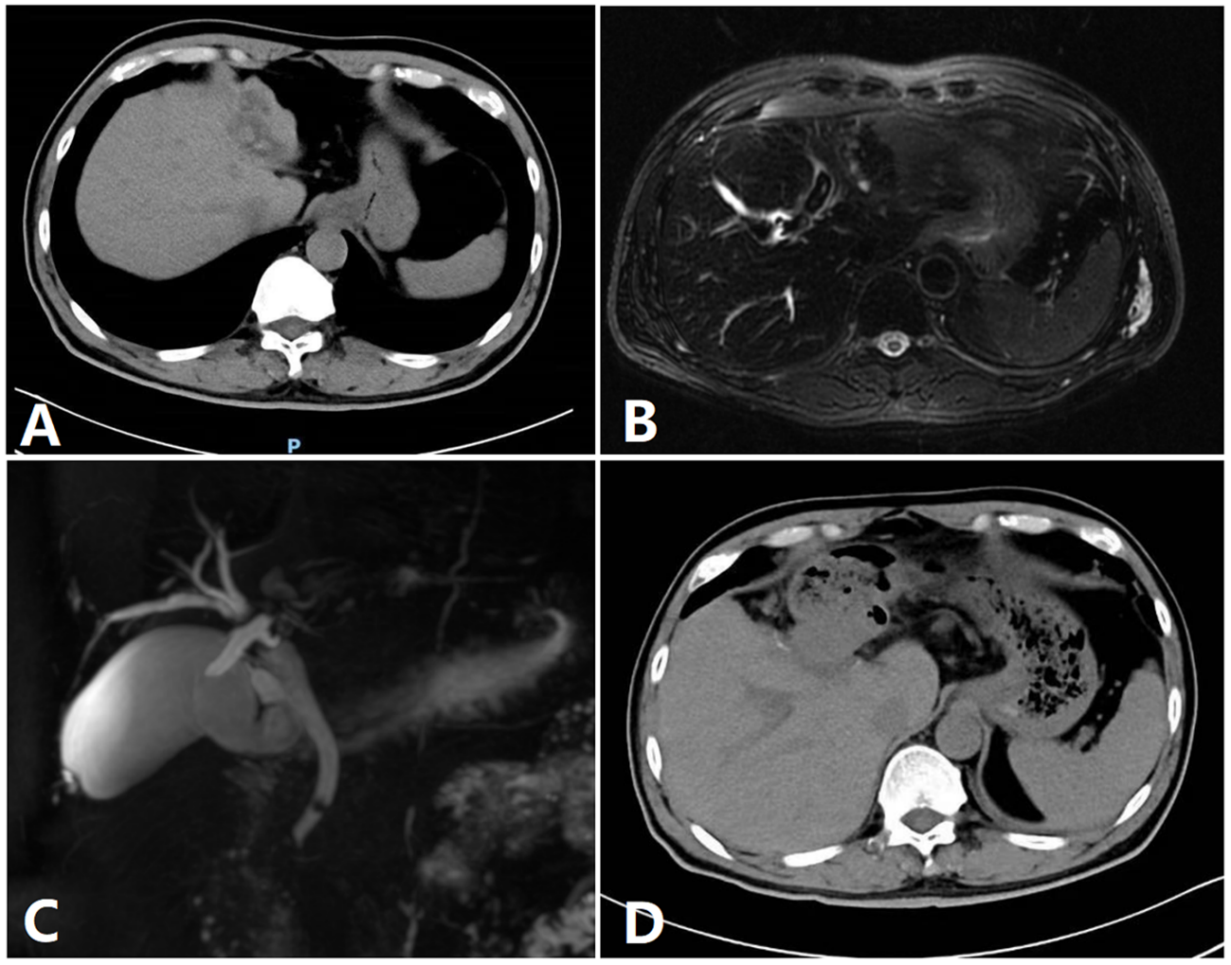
Image of a patient. (A) A preoperative CT image of a patient. (B) Preoperative MRI. (C) Preoperative bile duct water imaging. (D) CT imaging after 1 week.
